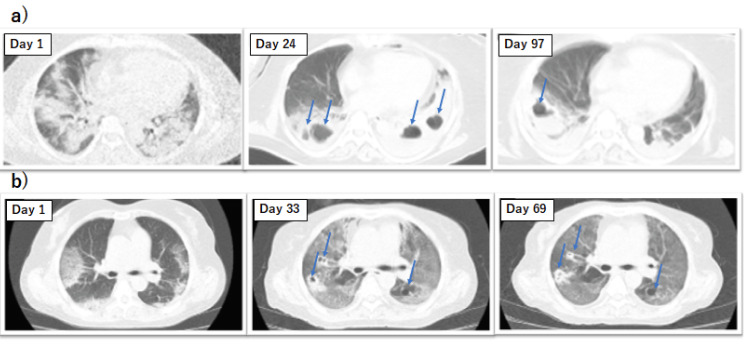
Serial chest CT findings a) Case 1: the bilateral cavitary lesions (arrows), one of which was recognized by X-ray on day 16, were recognized by CT on day 24. After the administration of ITCZ from day 58, the cavitary lesions diminished on day 97 b) Case 2: the bilateral cavitary lesions (arrows) were recognized for the first time on day 33. After the administration of ITCZ from day 35, the cavitary lesions diminished on day 69 CT: computed tomography; ITCZ: itraconazole
